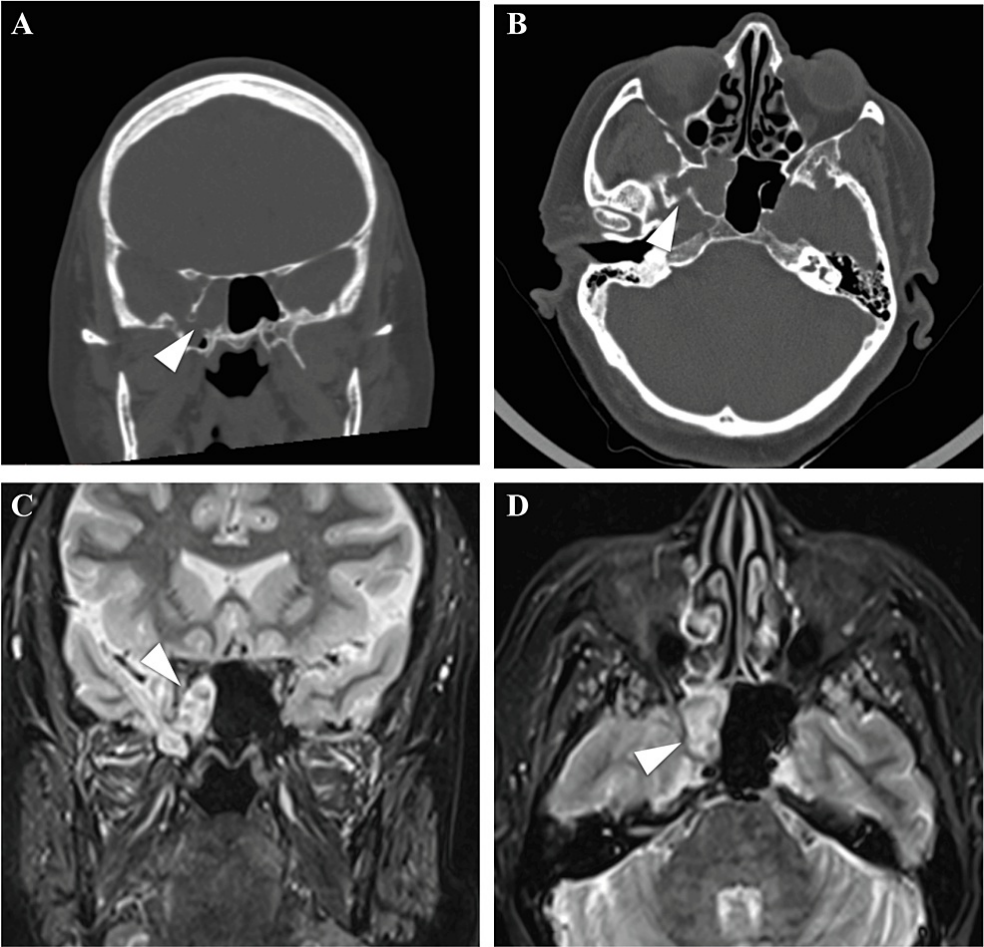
CT scans and MRI confirm inferolateral sphenoid wall dehiscence and brain herniation. CT scans (A and B) show a dehiscence of the inferolateral sphenoid wall, establishing communication with the middle cranial fossa (arrowheads). MRI (C and D) shows bone discontinuity in the right lateral wall of the sphenoid sinus and in the ipsilateral petrous apex, crossed by tissue with signal emission characteristics similar to brain parenchyma and CSF, involving the right chamber of the sphenoid sinus, the ipsilateral Meckel cave, and the base of the pterygoid processes bilaterally (arrowheads).
